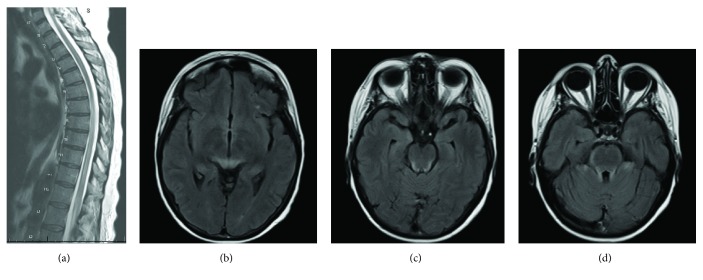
MRI images of severe IT chemotherapy complications.* (a) Increased T2 signal throughout the spinal cord, most prominent from T6 to the conus. There is some associated cord expansion. The cord signal involves almost the entire diameter most noted at T8. (b-d) Brain images showing symmetric FLAIR signal abnormality in the brainstem, cerebellum, and possibly the thalami without diffusion restriction or abnormal enhancement.
